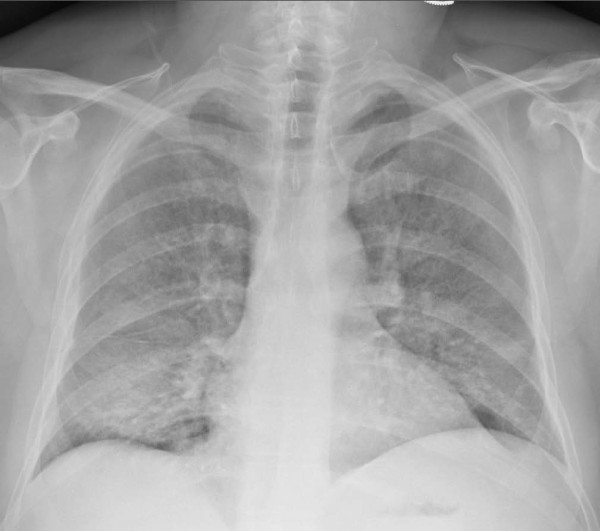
Chest radiograph. Chest X-ray showing bilateral airspace disease.
